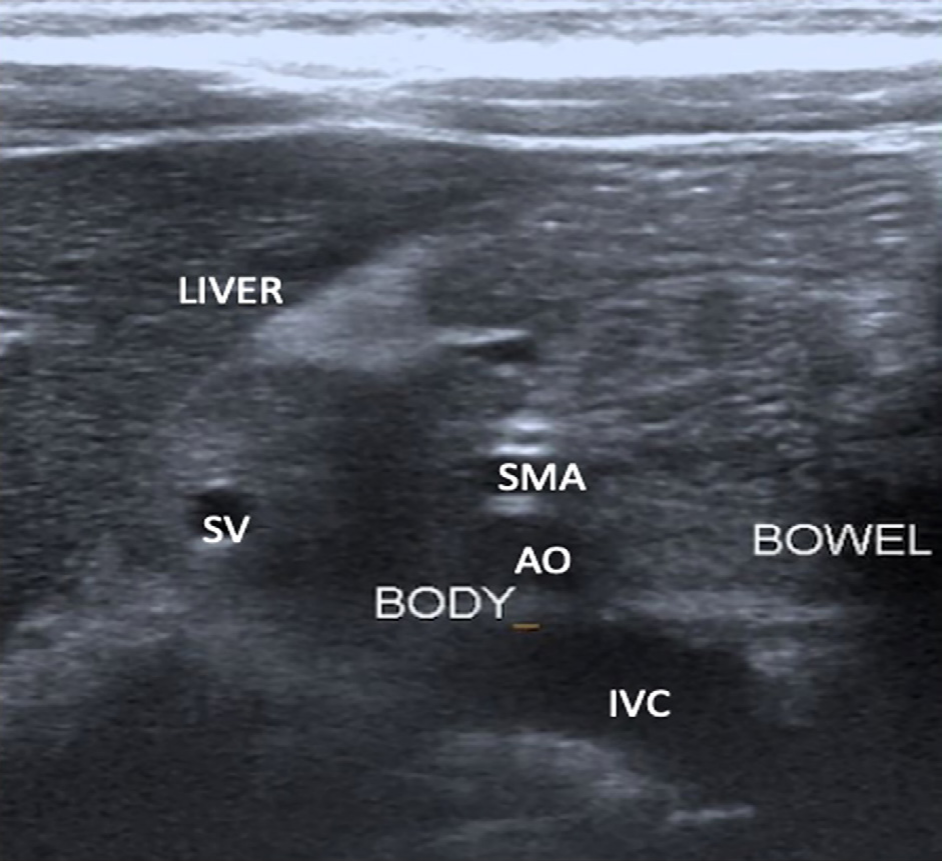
Transabdominal ultrasound midsagittal plane showing normal body and head of pancreas. SV: splenic vein, AO: abdominal aorta, SMA: superior mesenteric artery, IVC: inferior vena cava.
